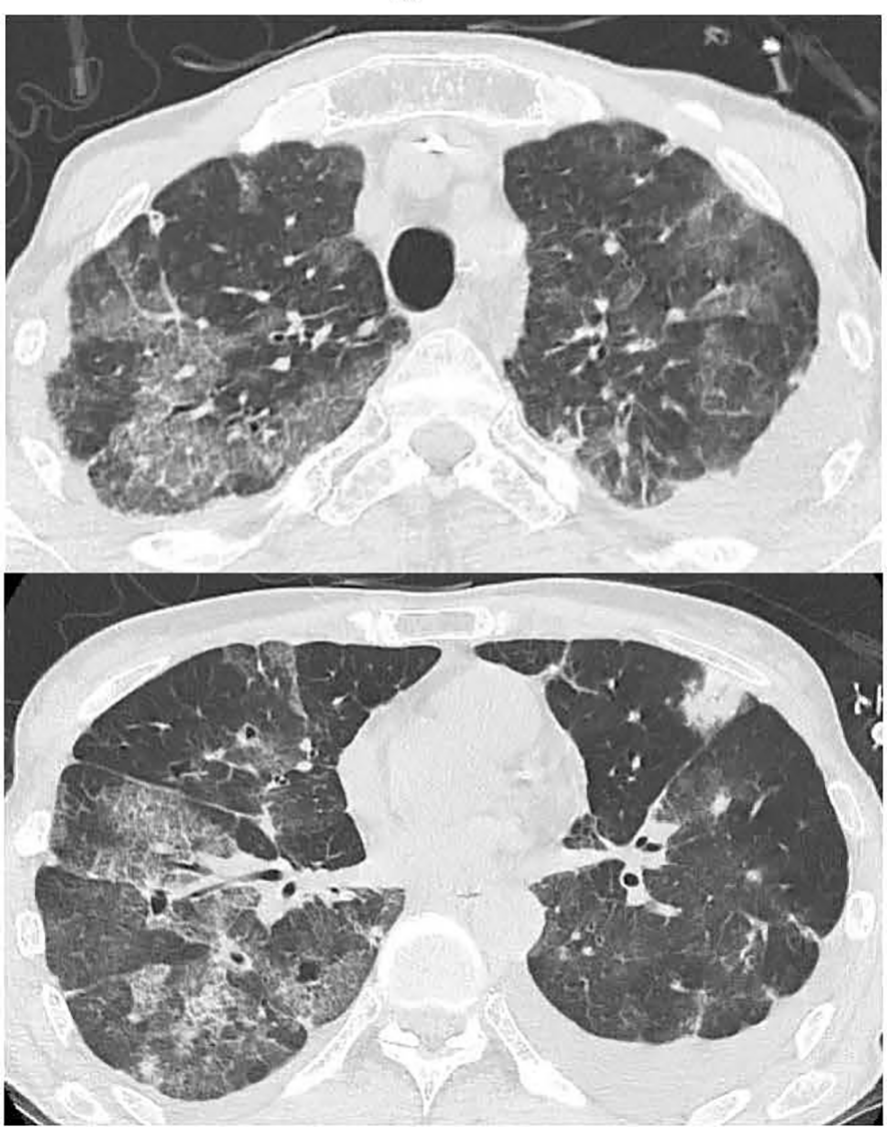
Chest CT showing exacerbation of bilateral lung interstitial infiltrate shadows on day 6 after admission.
